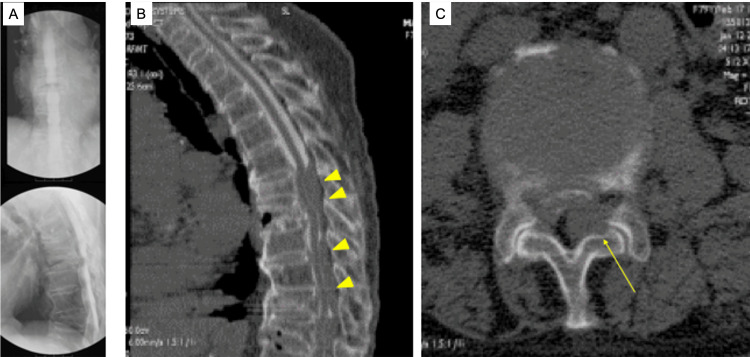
Radiographic findings in Case 1 after myelography. (A) Lateral X-ray during myelography showing dorsal pooling of contrast at the thoracic level. (B) Sagittal CT myelography demonstrating a dorsal contrast defect extending from T7 to T12 (yellow arrowhead). (C) Axial CT myelography image showing dorsal compression of the thecal sac by an epidural mass (yellow arrow) CT: computed tomography
